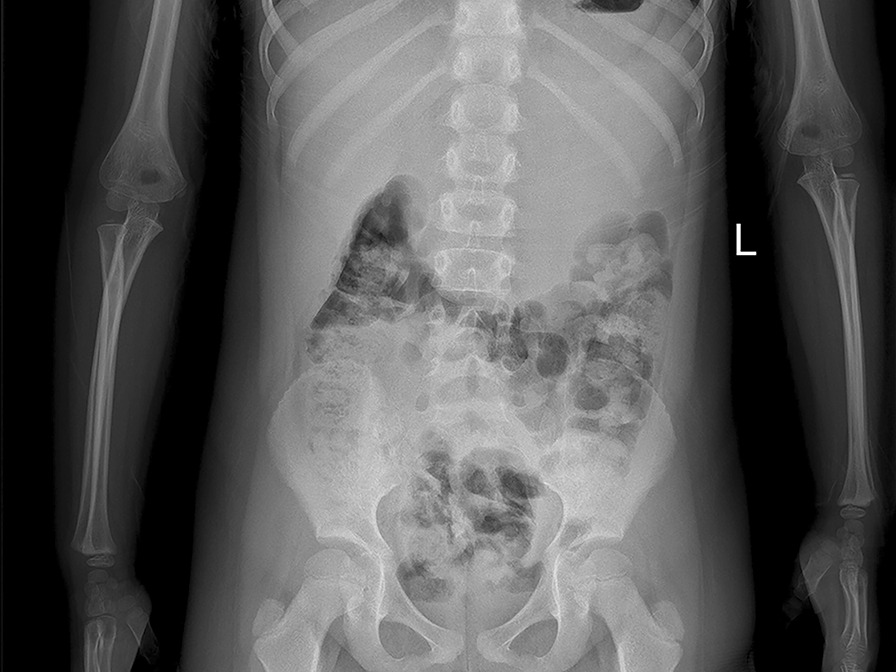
Digital radiography shows obvious accumulation of gases in the colon and no obvious abnormalities in the rest of the intestines
